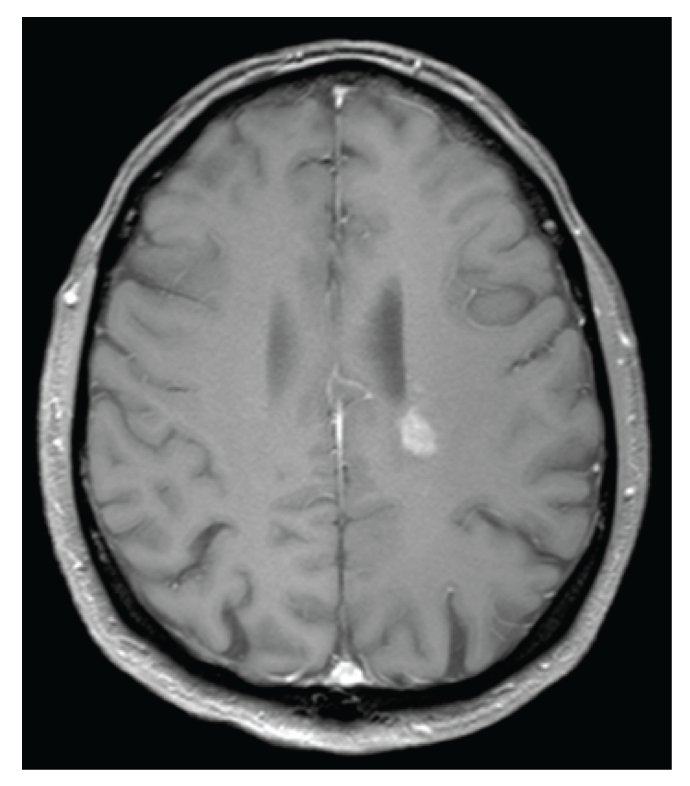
Axial postcontrast T1-weighted brain MRI shows a contrast-enhanced left-sided lesion in the periventricular white matter.
